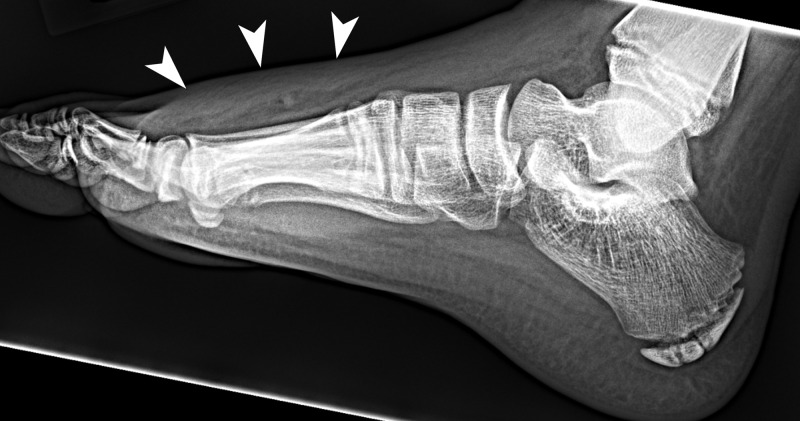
Lateral radiograph of the right foot Initial lateral radiograph of the right foot shows focal soft tissue swelling (arrowheads) overlying the dorsum of the foot but no radiopaque foreign body.
